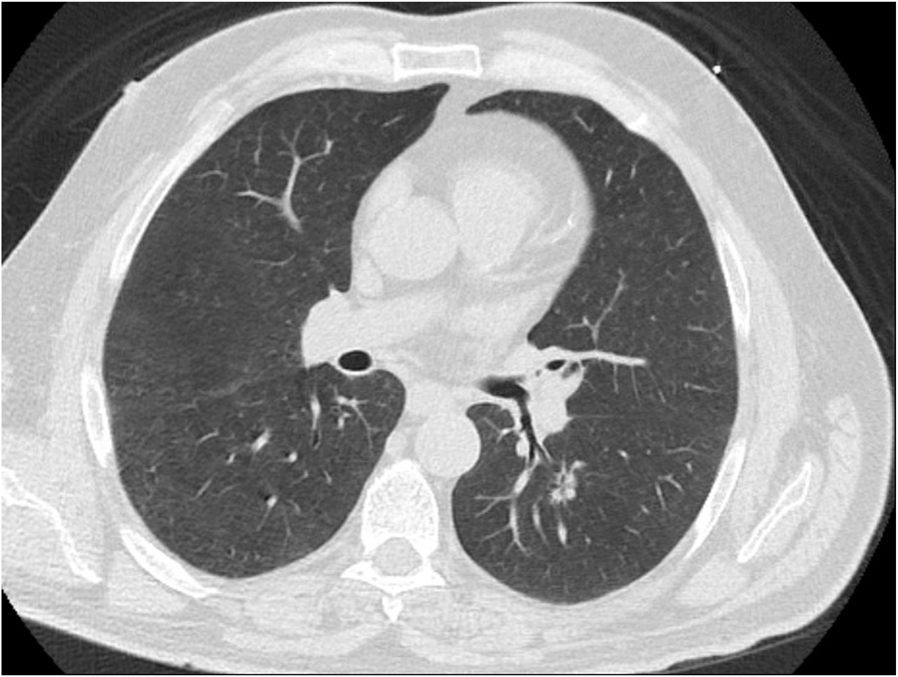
Repeat thoracic computed tomographic scan taken in July 2016
